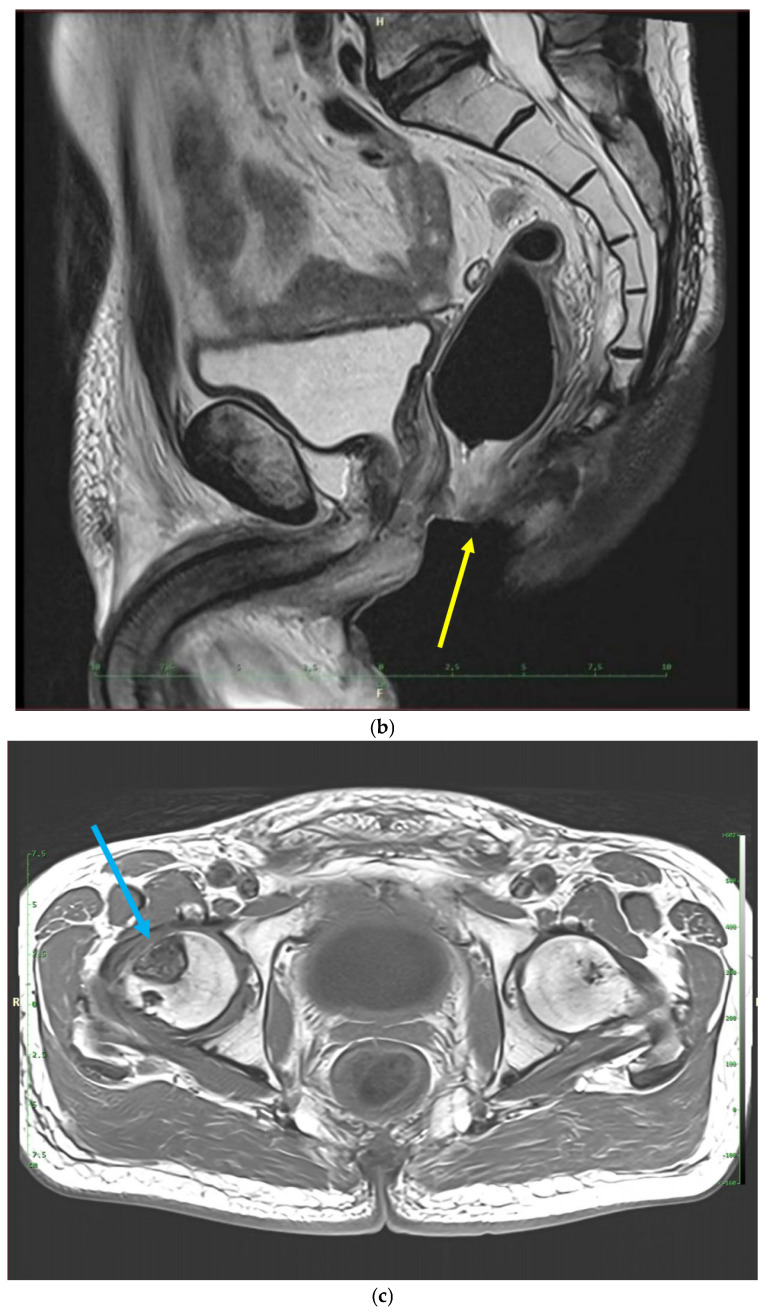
MRI scans 26 years after radiotherapy: T1-weighted axial MRI scan shows vast perineal and anal defects progressing to the left lower arm of the pubis with signs of induced osteomyelitis (orange arrow) (a); T2-weighted sagittal scan shows vast perineal and anal defects including absence of anal sphincter (yellow arrow) (b); and T1-tse weighted axial scans show the osteoradionecrosis of the right hip (blue arrow) (c).
